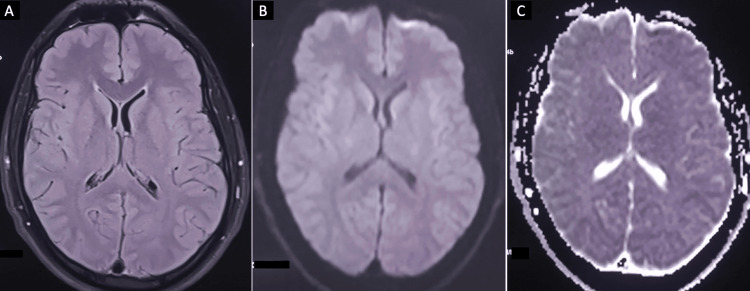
MR imaging findings on day 45, demonstrating the complete disappearance of previous abnormal intensity on FLAIR (A), DWI (B), and ADC map (C). FLAIR: Fluid-attenuated inversion recovery, DWI: Diffusion-weighted imaging, ADC: Apparent diffusion coefficient.
